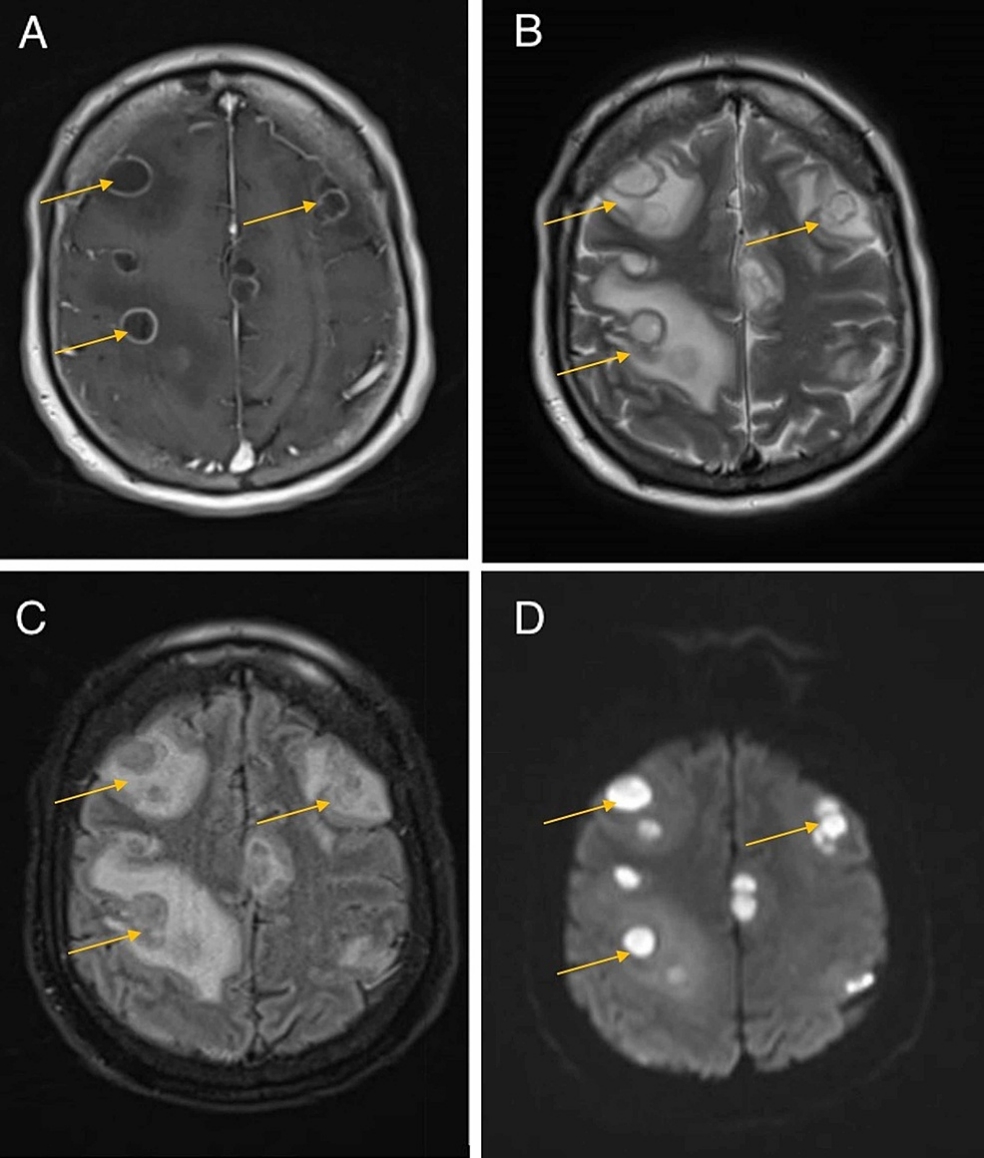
Initial MR brain imaging of the patient following new-onset tonic-clonic seizure (A) T1-weighted post-contrast imaging demonstrating multiple ring-enhancing lesions. (B) T2-weighted image demonstrating multiple hyperintense lesions with surrounding hyperintensity due to vasogenic edema. (C) T2-weighted-fluid-attenuated inversion recovery (T2-FLAIR) image demonstrating isointense signal within the lesions with surrounding hyperintensity due to vasogenic edema. (D) Diffusion-weighted imaging (DWI) restricted diffusion within the lesions consistent with an abscess.
